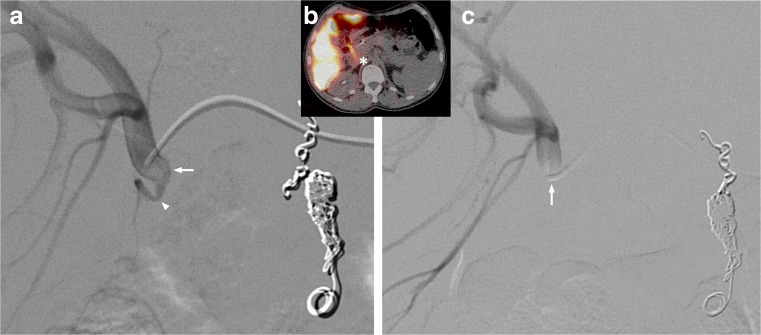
A 63-year-old male with colorectal cancer liver metastases. (a) Digital subtraction angiogram (DSA) of injection position of ^166^Ho scout dose procedure. (b) ^166^Ho scout dose SPECT/CT with extrahepatic deposition in duodenum (star). (c) DSA of injection position of ^166^Ho therapy. Note the difference in positioning of microcatheter (arrows). During ^166^Ho scout dose procedure the microcatheter pointed downwards, instead of horizontally (arrows). On the same DSA of ^166^Ho scout dose procedure the culprit vessel, supraduodenal artery, can be identified (arrowhead)
